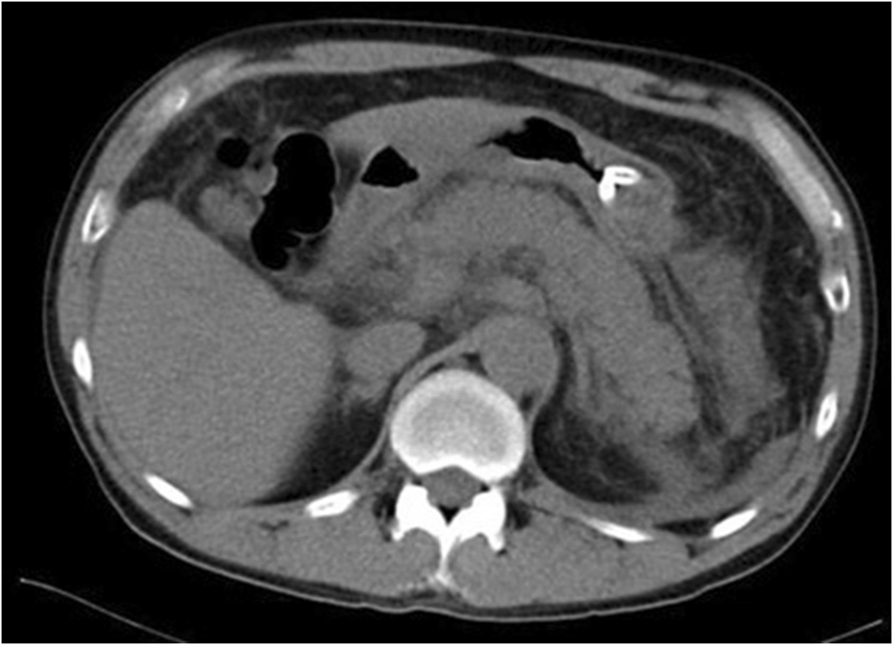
Abdominal computed tomography showing pancreatomegaly and an increased amount of peripancreatic fat.
